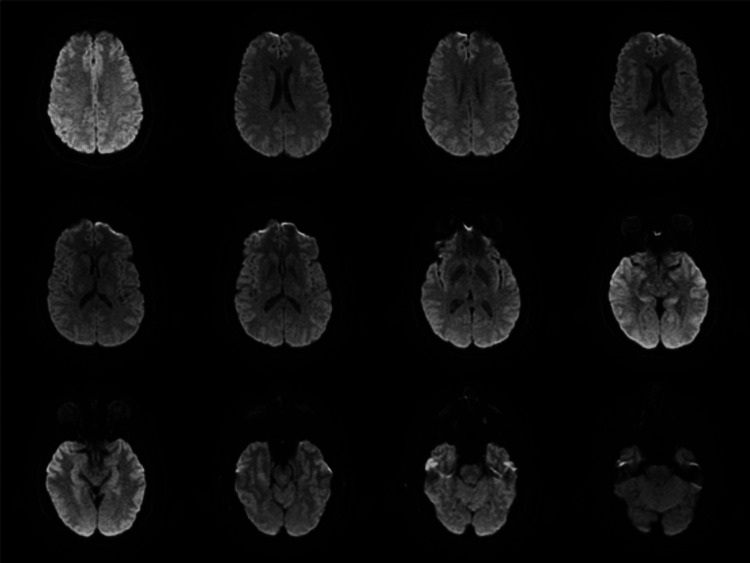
MRI brain without contrast Findings: The ventricular system is normal in size and configuration throughout. There is no hemorrhage, mass lesion, acute infarction or fluid collection. Normal signal flow voids are demonstrated within intracranial internal carotid and vertebrobasilar arteries. The sella, orbits, and visualized sinuses are grossly normal. Impression: No acute intracranial abnormality.
